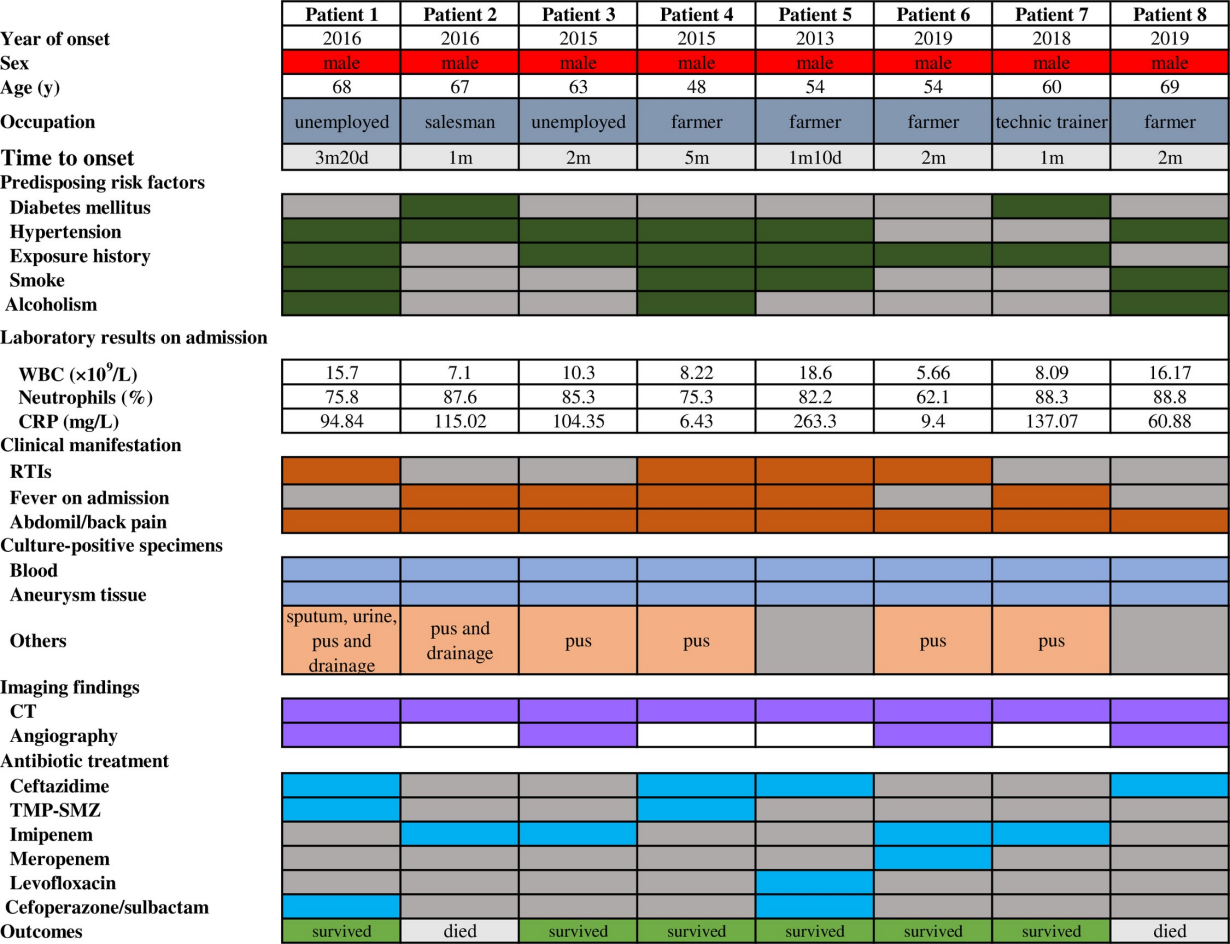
Clinical characteristics and epidemiological features of eight patients with mycotic aneurysms secondary to melioidosis in Hainan province, China: 2013–2019. RTI: Respiratory tract infections; CT: computed tomography; TMP-SMZ: trimethoprim/sulfamethoxazole; WBC: white blood cell; Blank cell: data not available; Grey cell: no; other colored cells: yes. Notes: in eight mycotic aneurysms secondary to melioidosis cases, all had positive detections in the CT scan of the abdomen and pelvis. However, by comparison, in 52 of bacteremic melioidosis without mycotic aneurysm, 44 had positive CT results.
